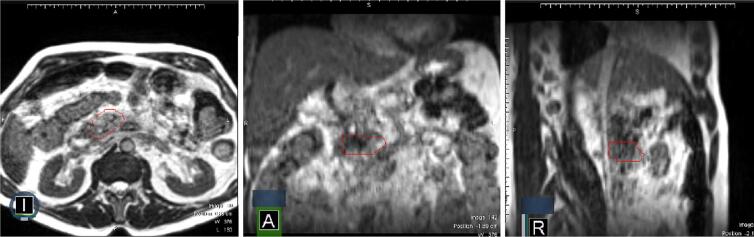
Example of patient images with 0.35 T MRI, GTV (ROI) delineated in red.
